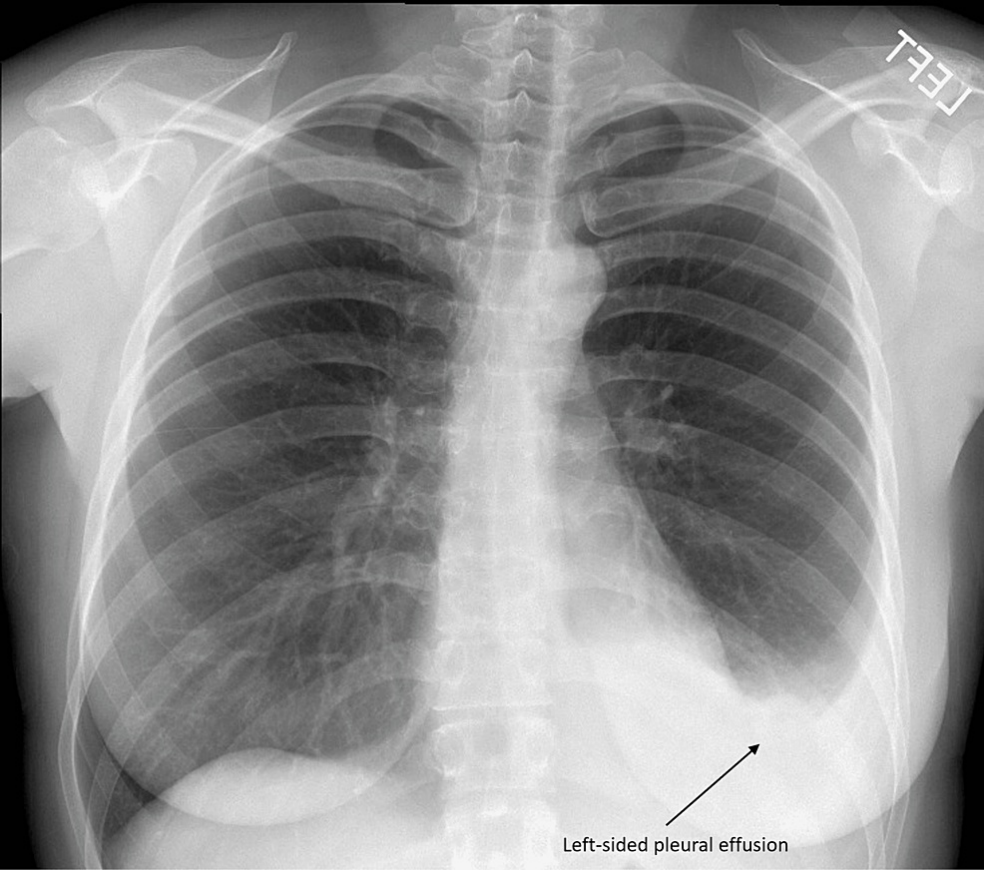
Chest X-ray demonstrating left-sided pleural effusion.
